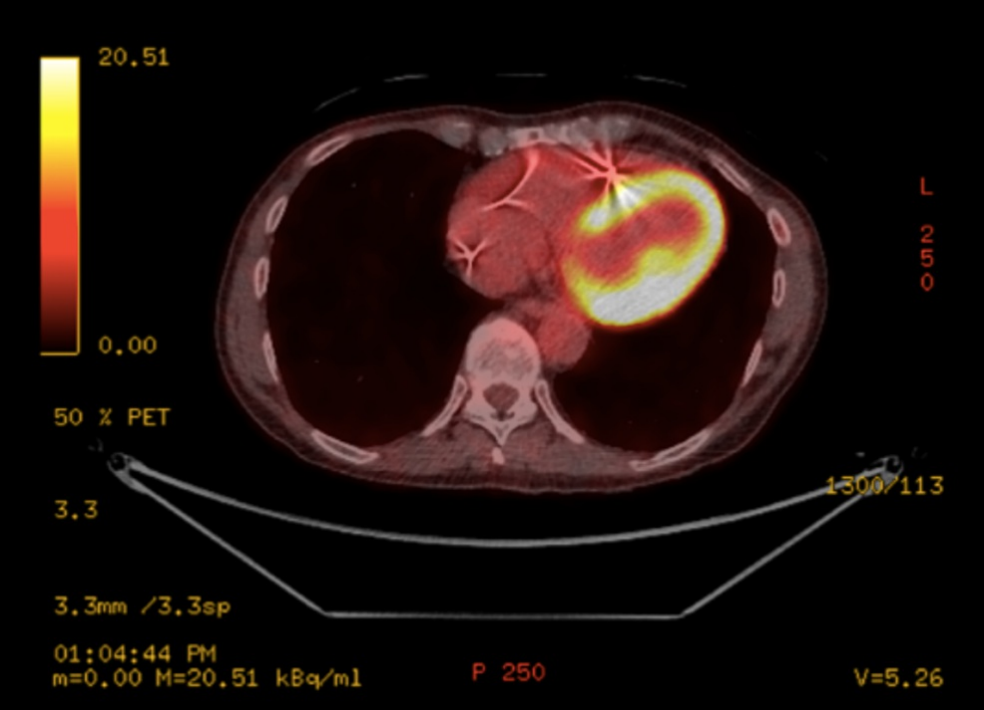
Reduced uptake of 18F-FDG in repeat PET/CT three months following discharge 18F-FDG: 18F-fluorodeoxyglucose; PET/CT: positron emission tomography/computed tomography
